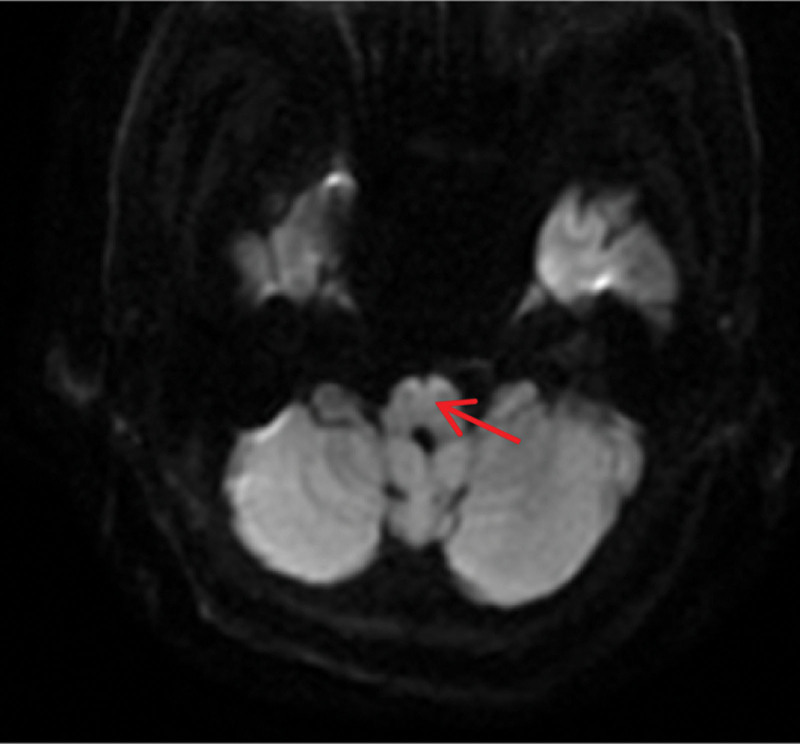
Axial DWI showed a hyperintense “heart appearance” signal in the bilateral anteromedial medulla (red arrow). DWI = diffusion weighted imaging.
